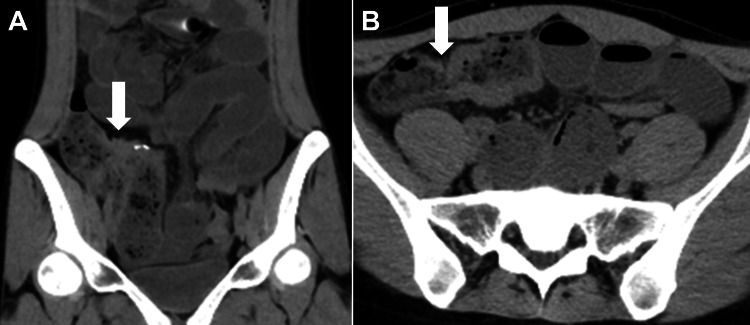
Abdominal computed tomography (A) Coronal computed tomography image showing proximal small bowel dilation and intraluminal fluid, with the postoperative anastomosis of the terminal ileum (white arrow) identified as the site of obstruction. (B) Axial computed tomography image showing small bowel wall thickening, with the postoperative anastomosis of the terminal ileum (white arrow) serving as the site of obstruction.
